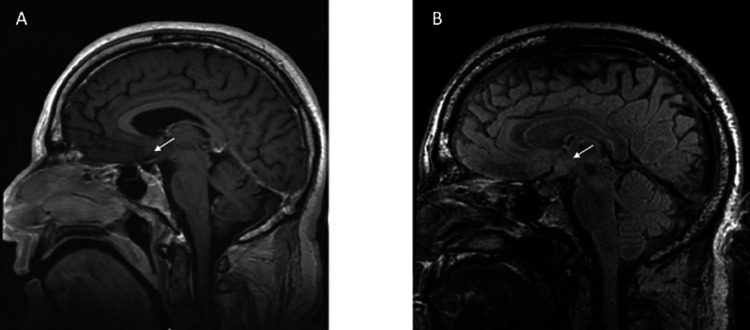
Subject 1 MRI brain at (A) 12 and (B) 27 months post-SRS treatment The white arrows show the area of lesioning in the subgenual cingulate. SRS, stereotactic radiosurgery
